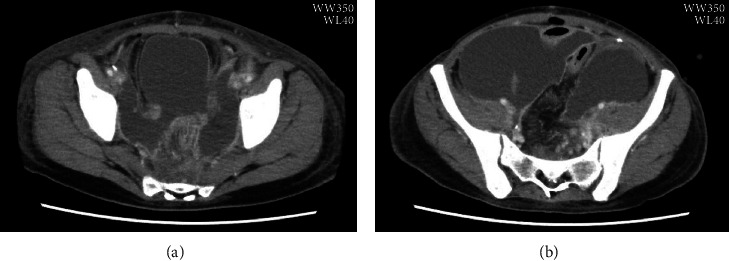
Computed tomography (CT) images on postoperative day 18. (a) Intestines are dilated, and the abdominal wall is thickened. Numerous septal walls can be seen in the abdominal cavity, where a large amount of ascitic fluid has accumulated. (b) The intestine is covered with a membranous capsule.
